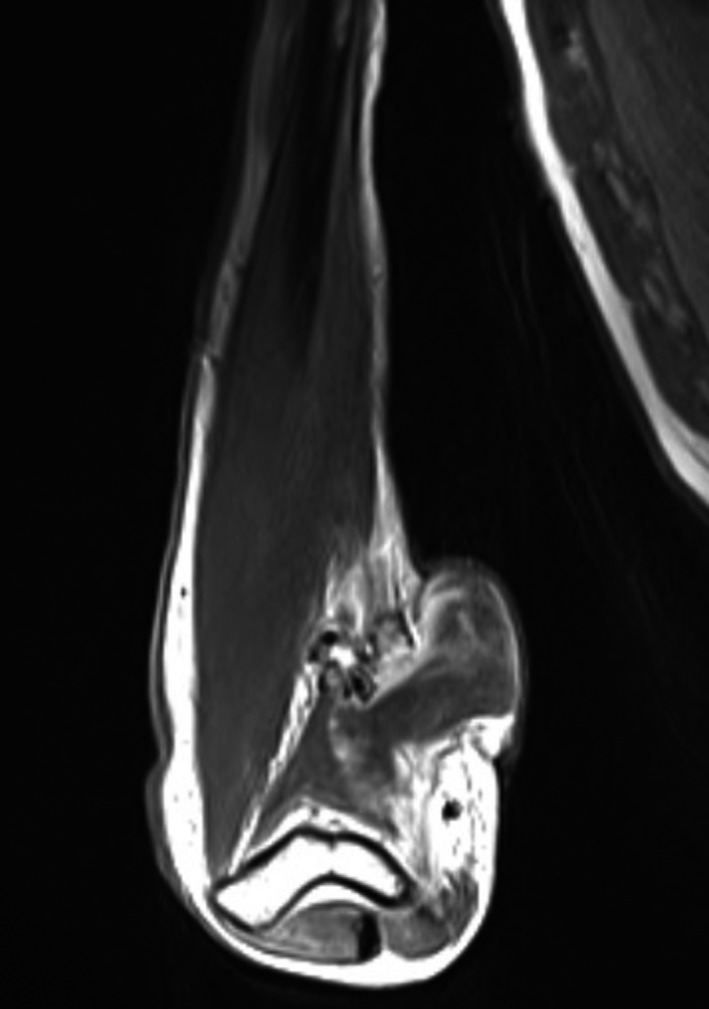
MRI, T1‐weighted image with contrast showing the patient's soft tissue mass with connection to the epidermis
